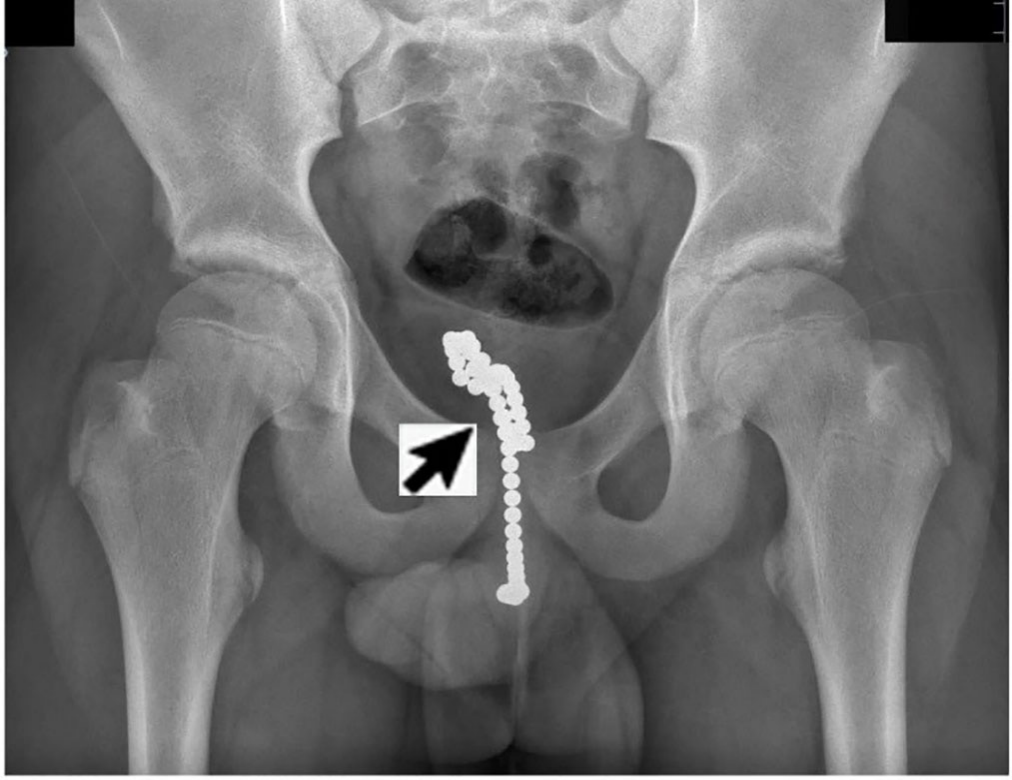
An anterior posterior plain film radiograph of the pelvis showing magnetic ball bearings in bladder and urethra.
